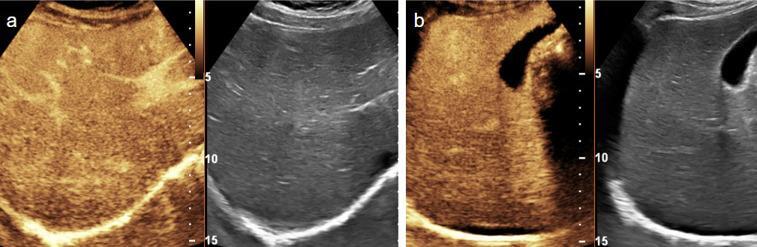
Motor vehicle collision in an 11-year-old girl. (a) Transverse and (b) sagittal contrast-enhanced ultrasound (CEUS) images with a dual display of contrast (left) and gray-scale (right) mode of the right lobe of liver. CEUS images reveal homogeneous enhancement of hepatic parenchyma with clearly defined hepatic vasculature during the portal-venous phase
